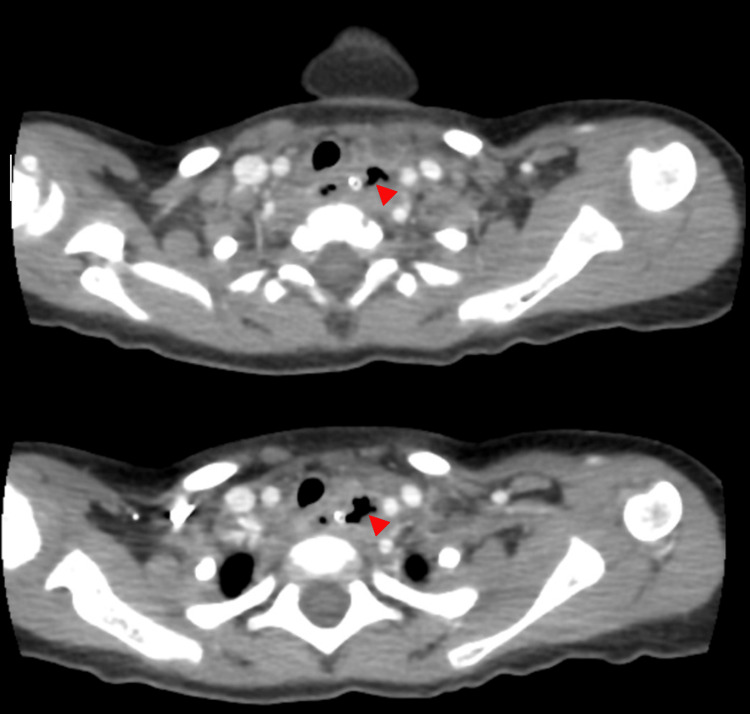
CT of the neck and chest The proximal part of the esophagus, inferior to the thyroid gland, shows an irregular configuration with extraluminal air foci (arrowhead) and rim enhancement, suggestive of focal esophageal perforation. There were no signs of blood vessel injury at the level of the study. CT: computed tomography
